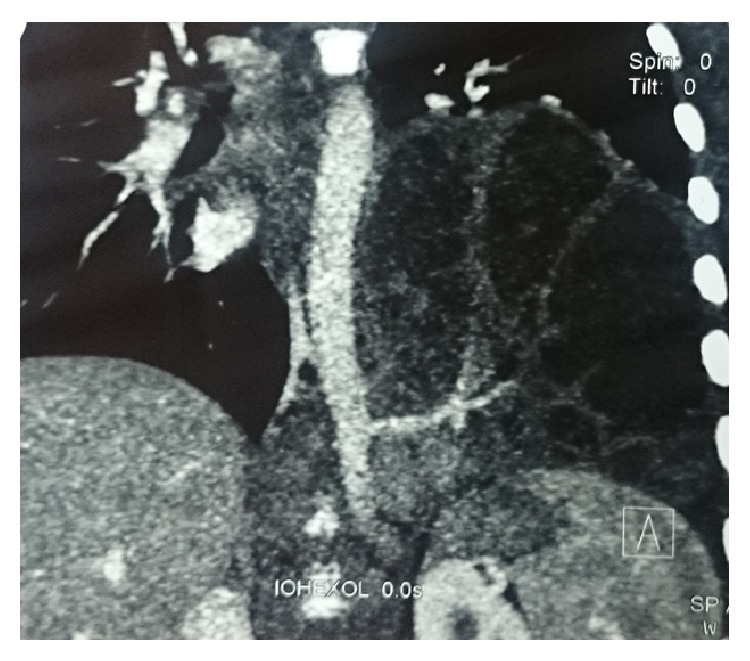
CT angiography shows intralobar lung sequestration with anomalous blood supply from the descending thoracic aorta.
